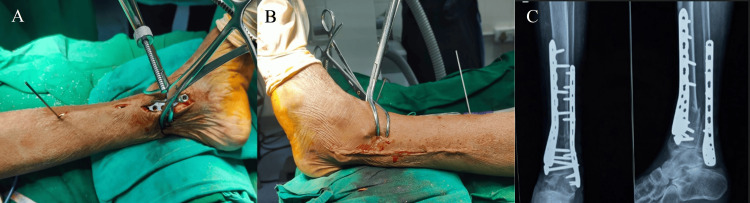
Intraoperative clinical and fluoroscopic images of MIPO tibial and ORIF fibular plating in closed distal tibial and fibular fractures (A) The technique of MIPO for the distal tibia involves indirect reduction using K wires and a reduction clamp. A sub-muscular plane is then created using a Cobb elevator, and a medial anatomical distal tibial locking plate is fixed. (B) The fibula is fixed using the standard ORIF technique. (C) Shows the postoperative radiograph. MIPO: minimally invasive plate osteosynthesis, ORIF: open reduction and internal fixation
